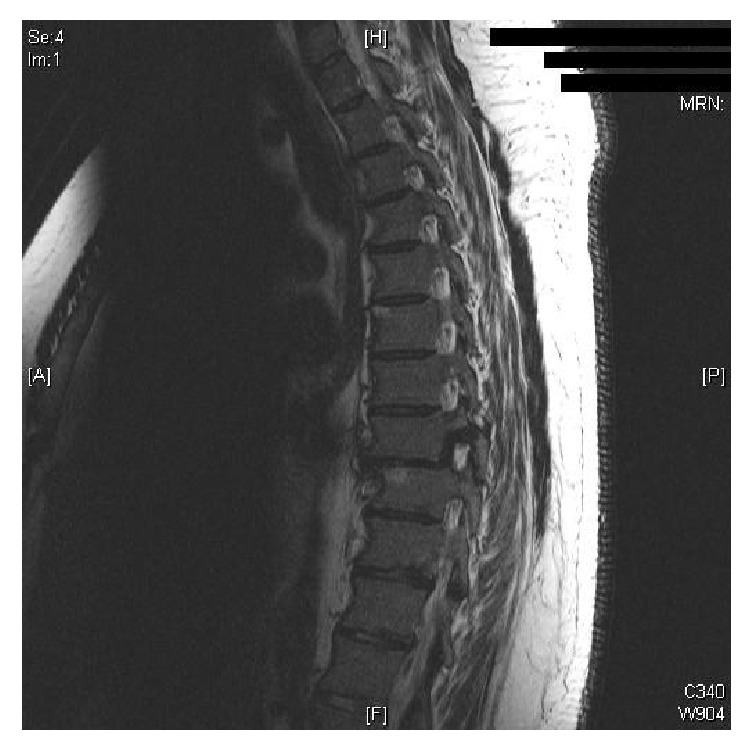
MRI of the thoracic spine at 10 weeks following initial presentation, showing a traumatic spondylolisthesis of T8 on T9.
